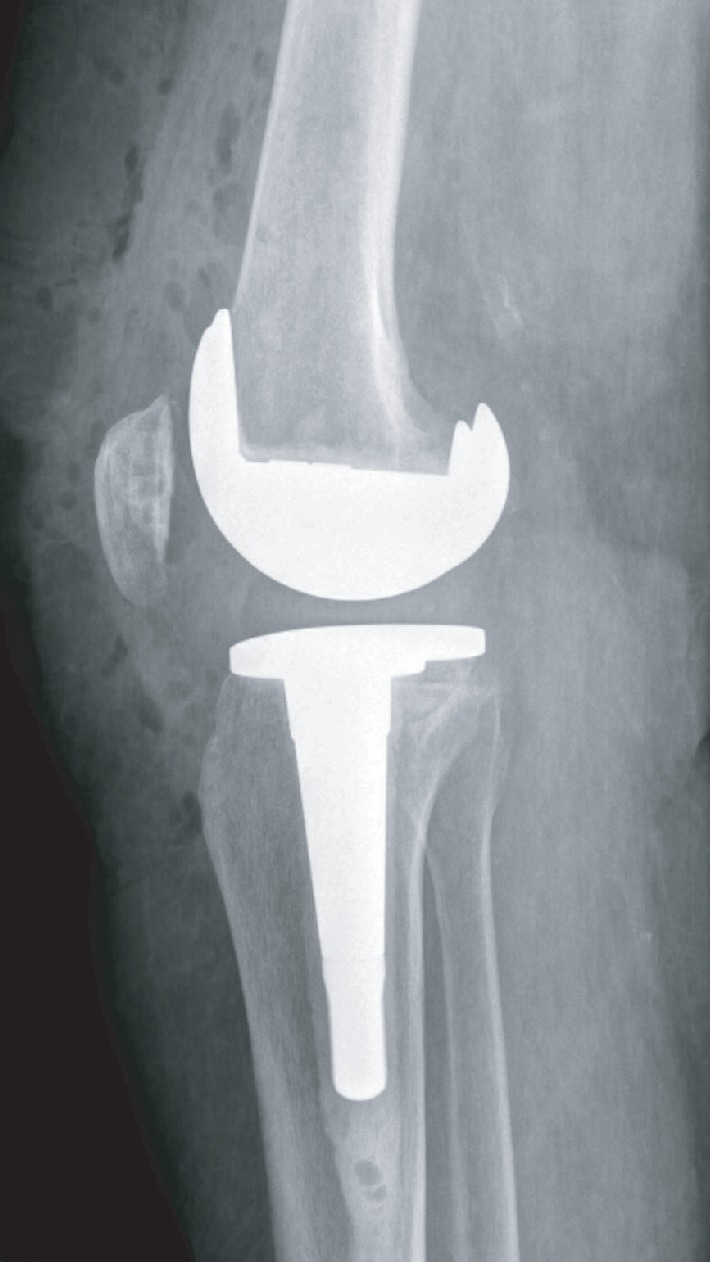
Postoperative lateral radiograph of the left knee. Revision left total knee in situ. Pin holes used for navigation are present distal to the stemmed tibial component.
